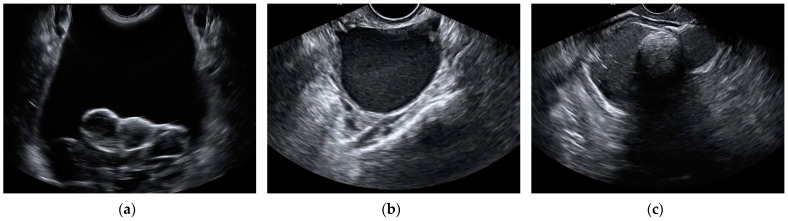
Still ultrasound images of benign ovarian masses misclassified as malignant by the aggregate model. (a) Serous cystadenoma, (b) endometrioma, and (c) mature cystic teratoma.
